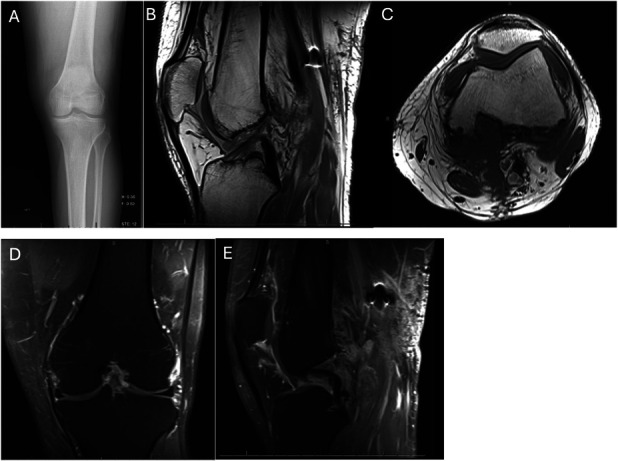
Postoperative imaging for patient after anterior arthroscopic and open posterior excision of tumor. Fig. 5-A Anterior posterior radiograph of the knee with only notable changes from preoperative imaging being curetting and bone grafting of the medial condylar defect. Fig. 5-B T1-sagittal and axial (Fig. 5-C) MRI demonstrating near complete disease resection with bone grafting of the posterior condylar defect. Fig. 5-D Coronal T2 and sagittal postcontrast (Fig. 5-E) MRI highlighting removal of the anterior and posterior intra- and extra-articular disease burden. MRI = magnetic resonance imaging.
